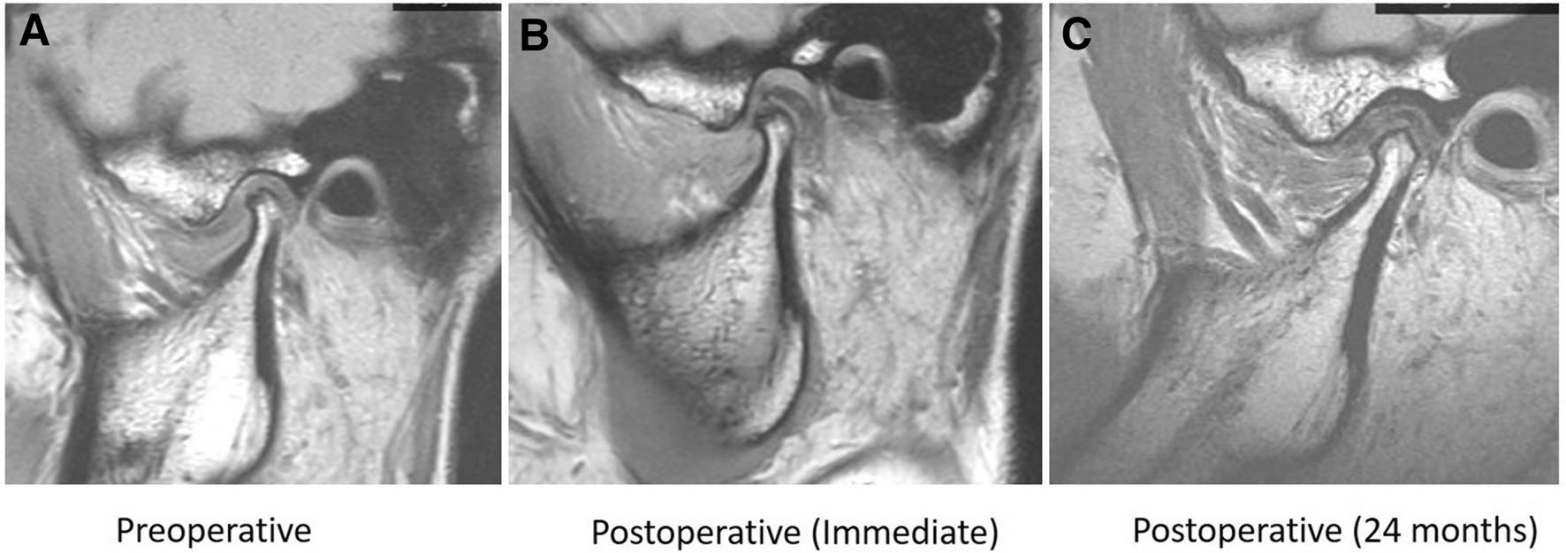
MRI scans of a 17-year-old female patient with ADD on the left side. (A) Preoperative MRI image, (B) Immediate postoperative MRI image showing successful disc repositioning, (C) Stable disc position with condylar bone remodeling at 24 months of postoperative follow-up.
